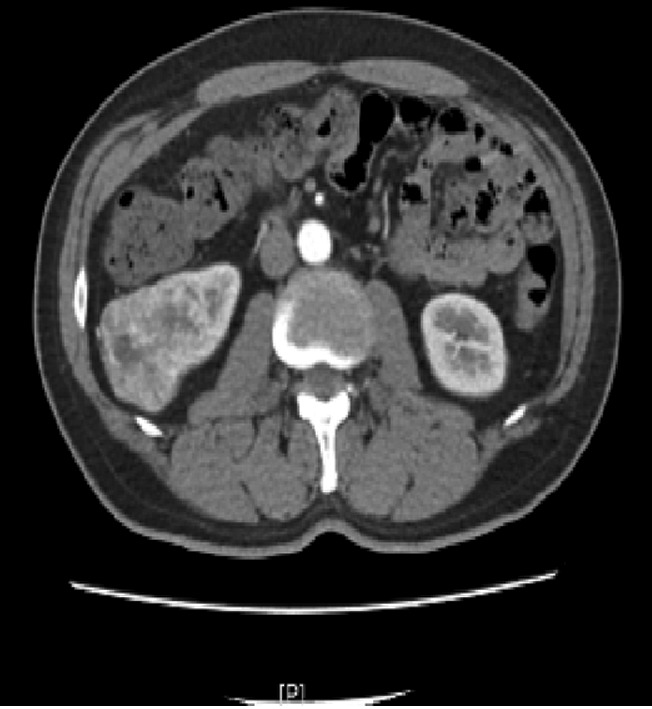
Pre-operative computed tomography image of patient 2, showing a mass in the middle-lower pole of the right kidney, extending out of the profile of the kidney. The renal fascia adjacent to the mass is thickened indicating a renal carcinoma.
